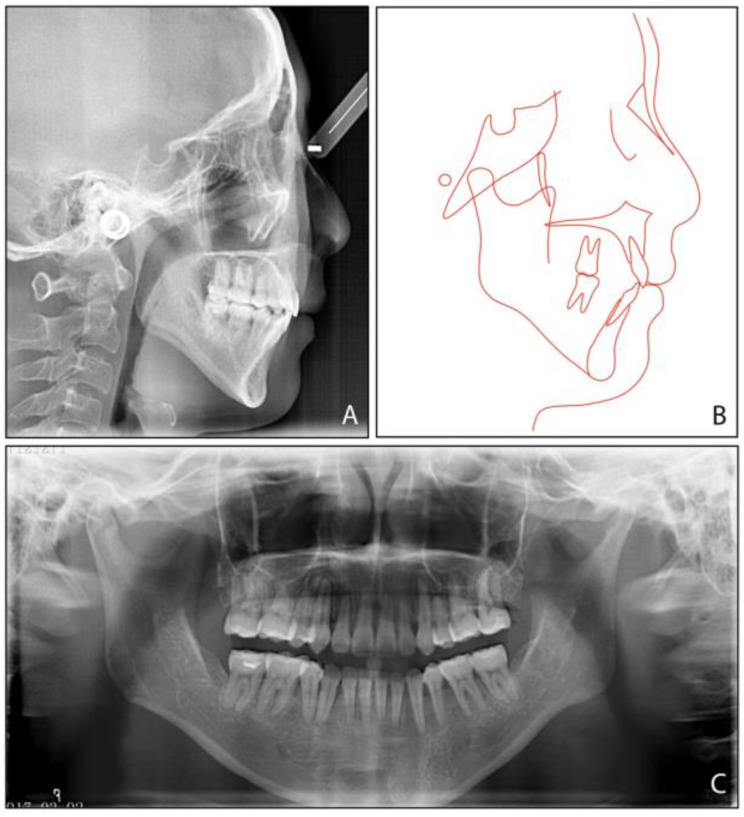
Post-treatment radiographs and tracing: (A) cephalometric radiograph; (B) cephalometric tracing; (C) panoramic radiograph.
